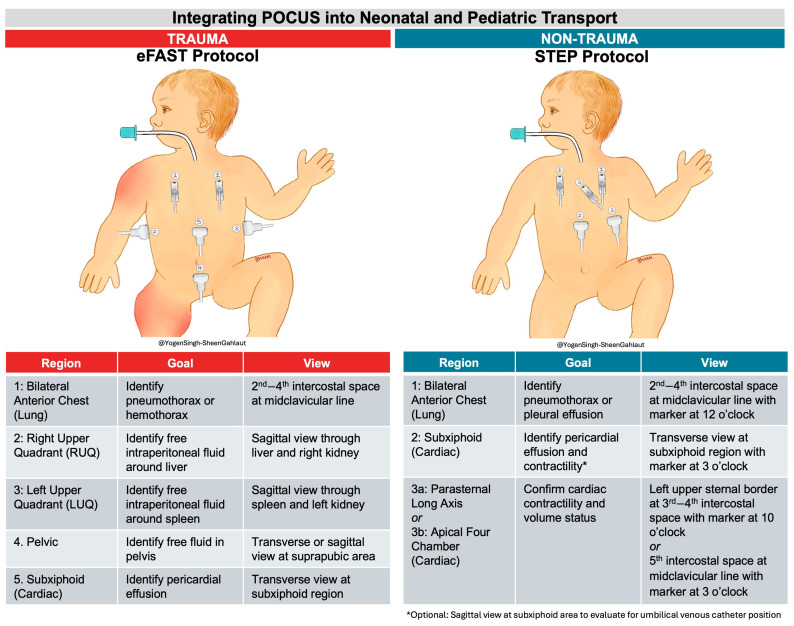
Neonatal and pediatric transport protocols, including eFAST (extended Focused Assessment with Sonography in Trauma) and our proposed protocol, STEP (Structured Transport Emergency POCUS), based on clinical indications.
